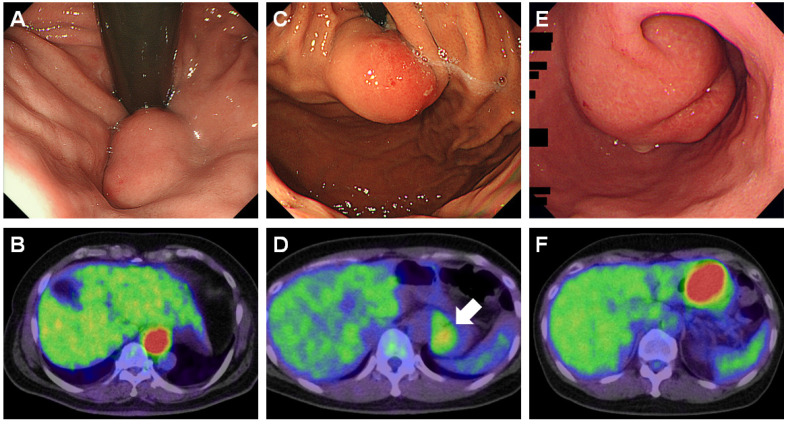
Representative endoscopic and 18F-fluorodeoxyglucose (FDG)-positron emission tomography images of gastric gastrointestinal stromal tumor (GIST), leiomyoma, and schwannoma. High-grade GIST that presented as a subepithelial tumor in the gastric cardia (A) and showed increased FDG uptake (B, maximum standardized uptake value [SUVmax] = 11.4, tumor size: 4.6 cm). A gastric leiomyoma was observed as a subepithelial lesion in the cardia (C). The SUVmax of the leiomyoma was 2.75 (D, tumor size: 2.1 cm). A schwannoma in the gastric body (E) showed increased tracer accumulation (F, SUVmax = 9.01, tumor size: 5.5 cm).
